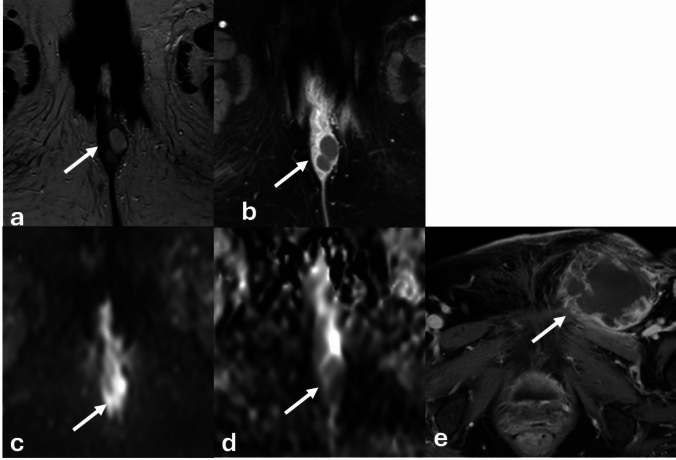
51-Year-old female with vulvar and left groin masses. Axial non-fat saturated (a), T1 post-contrast (b, e) and diffusion weighted MR images of the pelvis with accompanying ADC map (c-d) demonstrate an ovoid, uniformly enhancing T2 hypointense vulvar mass (arrow) with central cystic component. The lesion demonstrates diffusion restriction. Bulky, necrotic metastatic adenopathy is noted in the left groin (arrowhead)
